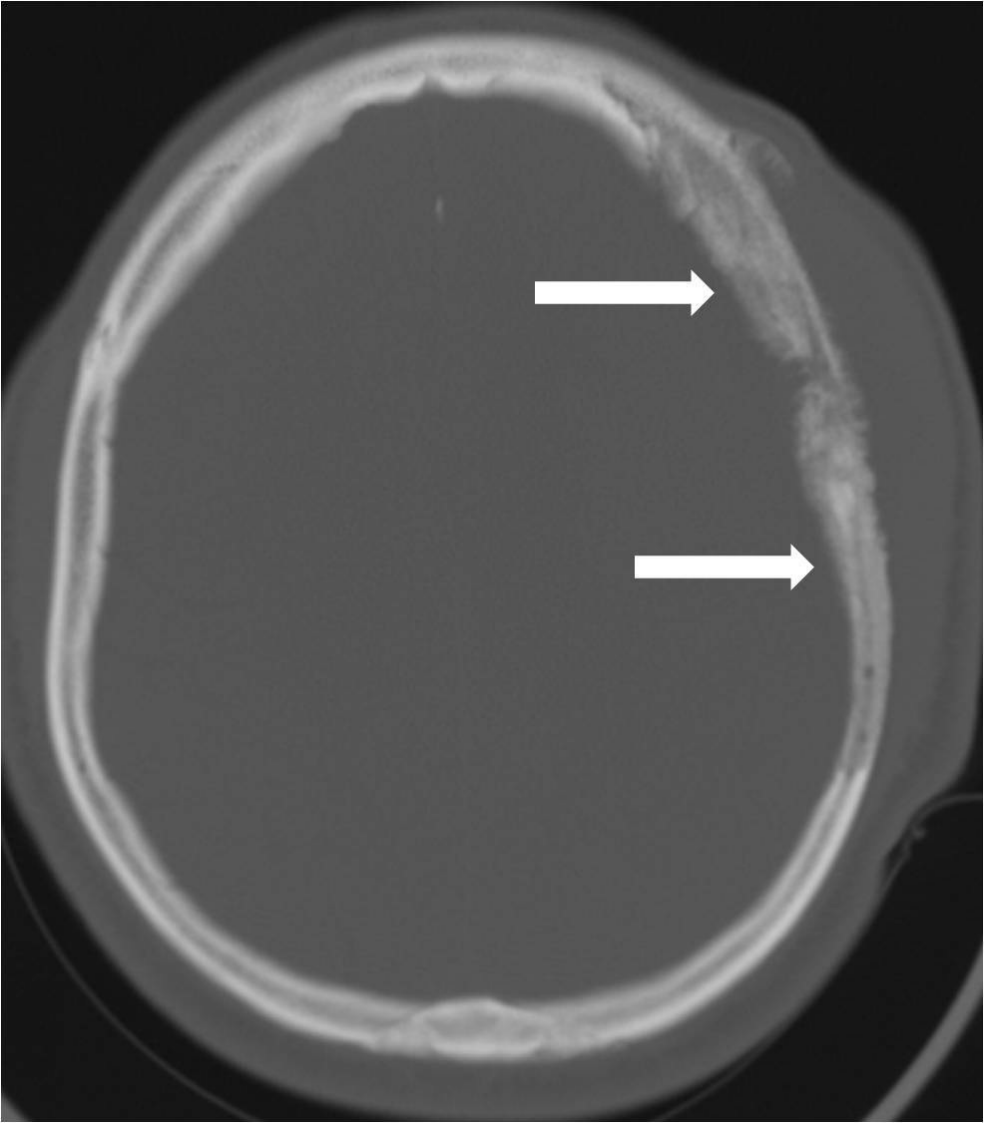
Bone windows from the head computed tomography (CT) reveal the osseous involvement with both osteolysis and hyperostosis of the left frontal and parietal calvaria (arrows).
